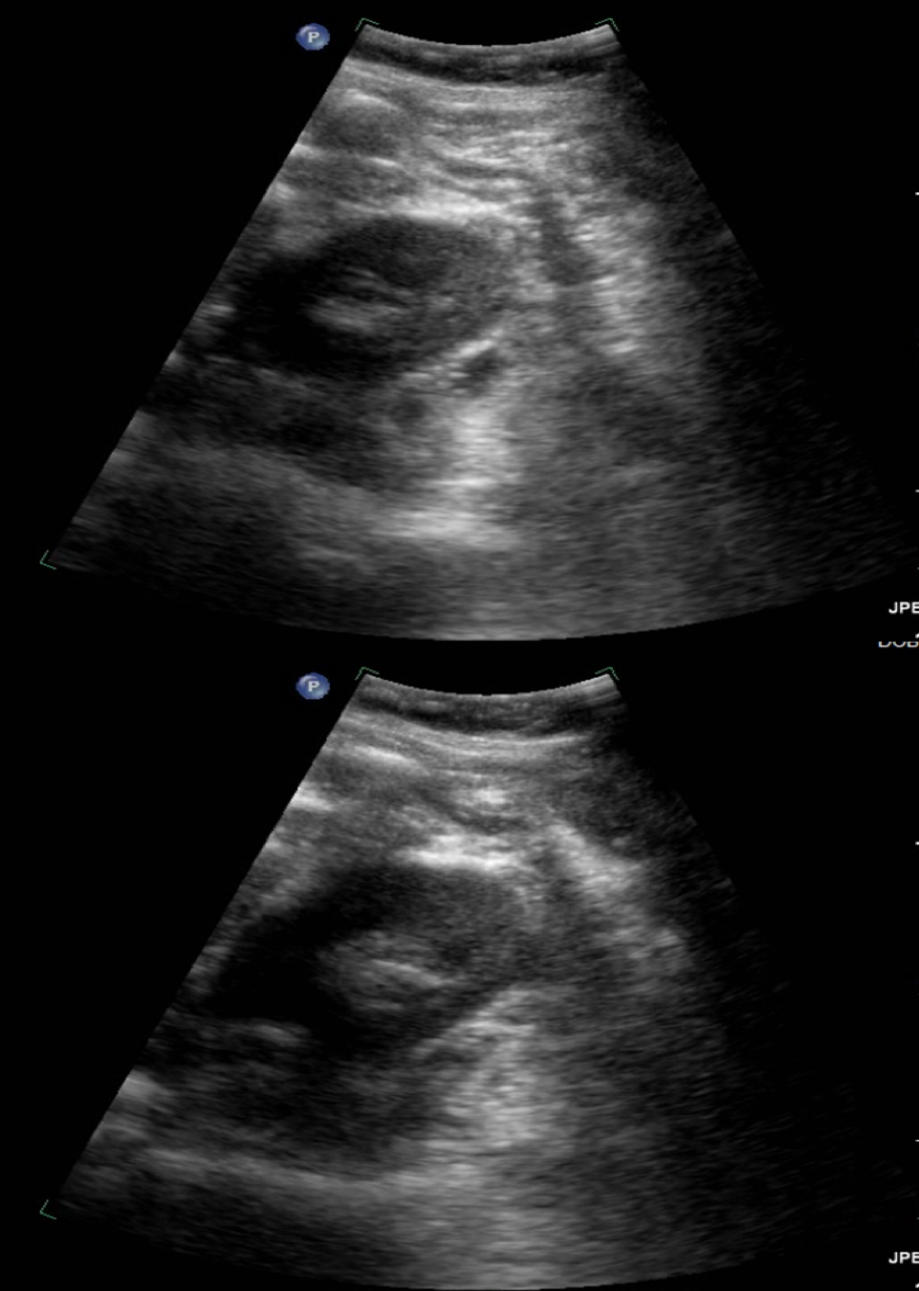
Ultrasonography Findings Ultrasonography findings reflect a heterogeneous lobular complex partially cystic lesion measuring approximately 2.8 x 2.4 x 1.6 cm with a soft-tissue component demonstrating internal vascularity in the subcutaneous soft tissues at the umbilicus.
